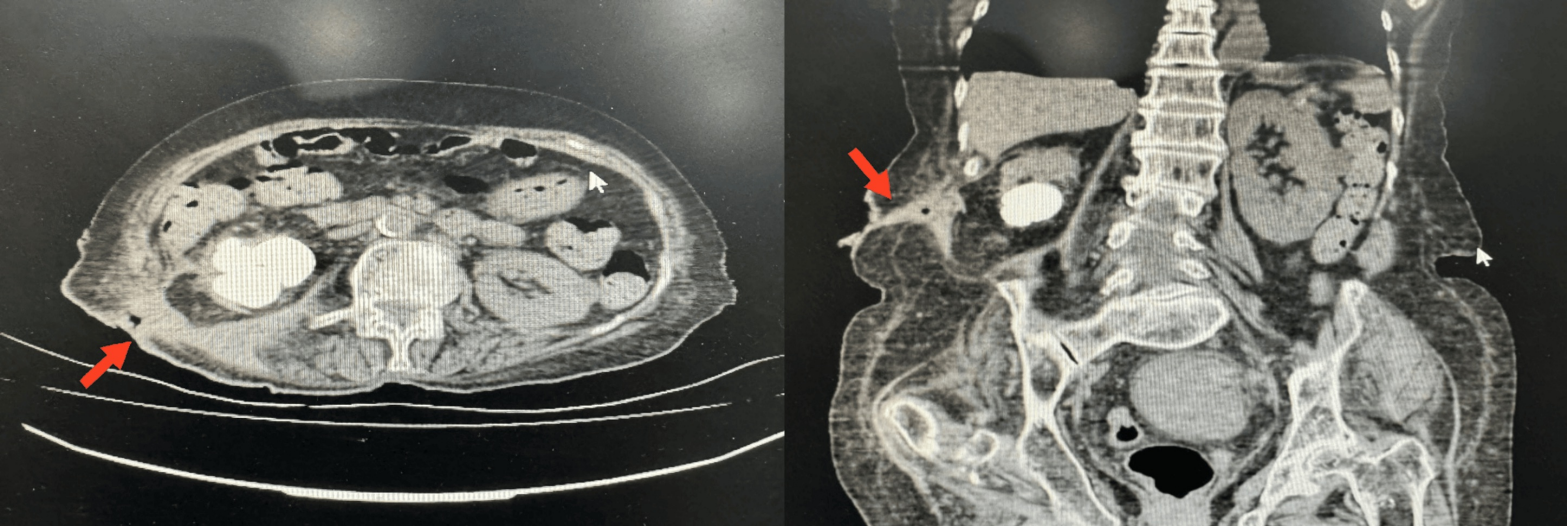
Axial (left) and coronal (right) CT scan sections at the level of the fistula. The axial section shows the extension of the fistulous tract from the right kidney to the skin. The coronal section gives a clearer view of the continuous fistula. The right kidney is shrunken with severe parenchymal thinning and multiple large calculi in the renal pelvis. Arrows indicate the location of the fistula in each image.
